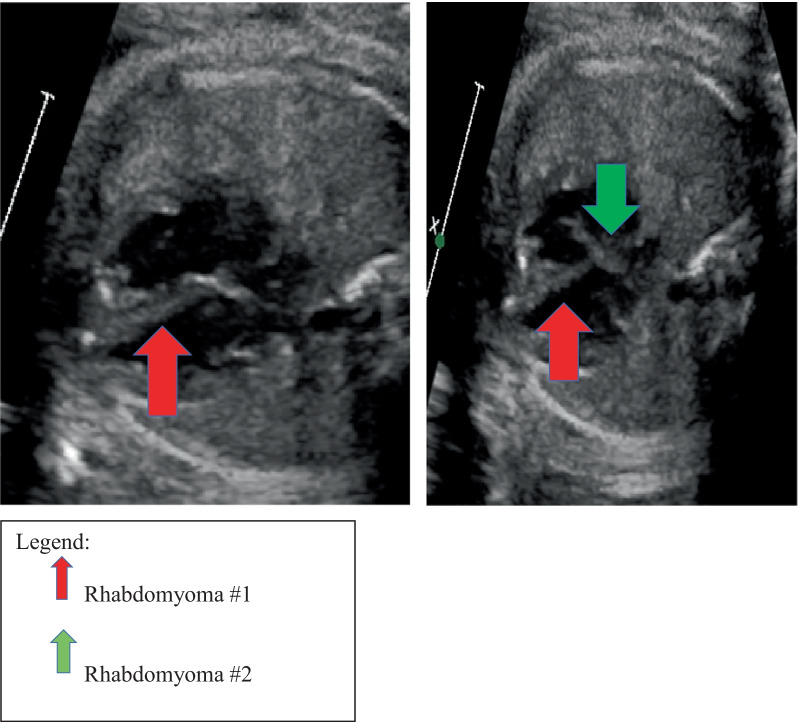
Fetal echocardiography at 32 weeks 5 days gestational age showed two similar small echogenicity in RV apex and septal aspect (4 mm × 2.6 mm) (arrows) suspicious of rhabdomyomas. Normal valvar function
